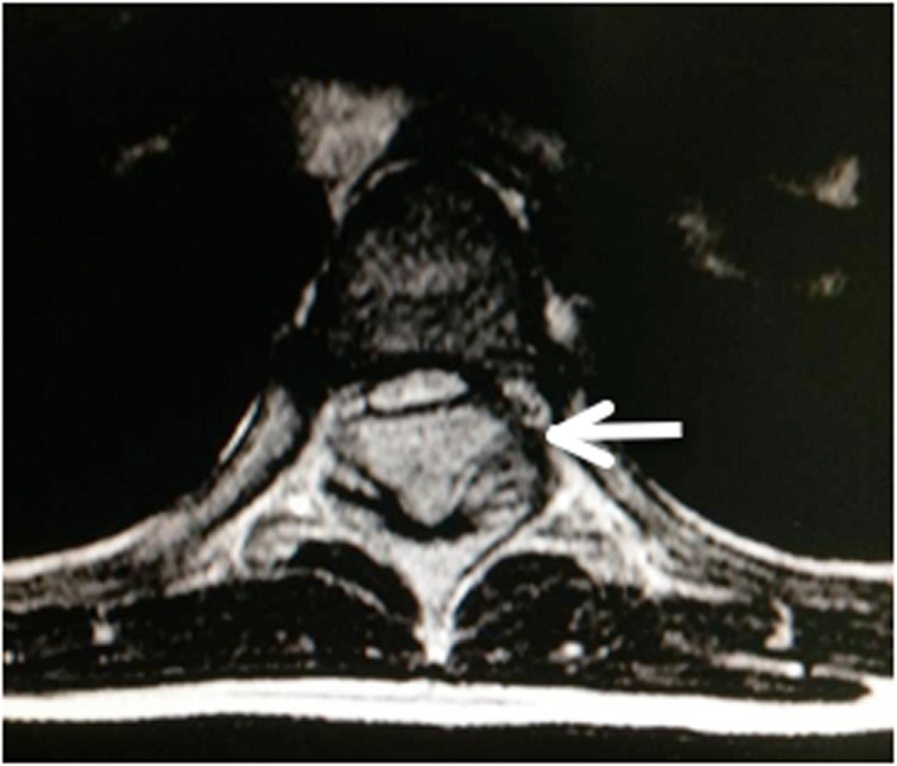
Preoperative axial magnetic resonance imaging. Axial T2-weighted image demonstrating tumor extension from the left facet joint of T5 to T6 (arrow).
